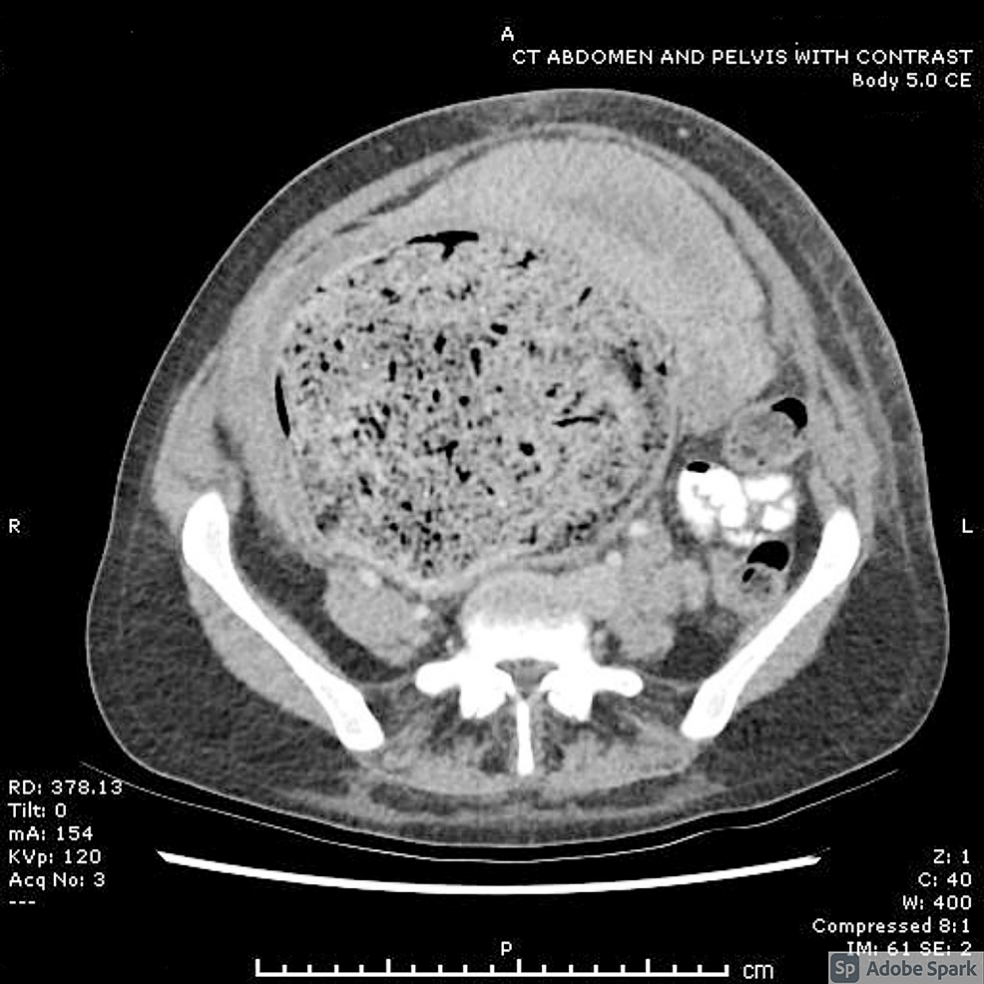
CT abdomen and pelvis - note uterus displaced by dilated bowel.
